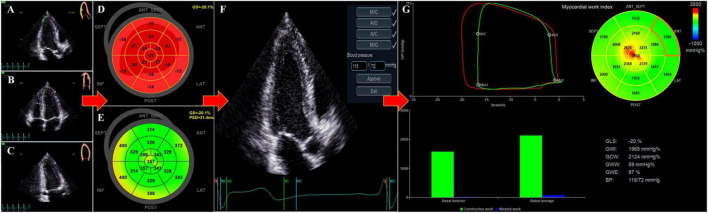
Non-invasive determination of left ventricular (LV) myocardial work from echocardiography. Using automated function imaging (AFI) software, images of the apical long–axis (A), apical four– (B) and two–chamber (C) views were analyzed to determine LV global longitudinal strain (GLS) (D) and peak strain dispersion (PSD) (E). After adjusting the event timing of aortic and mitral valve closure and opening in the apical long-axis view and inputting the blood pressure (BP) value (F), the Bull’s-eye images of 17 LV segments for myocardial work index or myocardial work efficiency were presented with global myocardial work values (G), including global work index (GWI), global constructive work (GCW), global wasted work (GWW), and global work efficiency (GWE).
